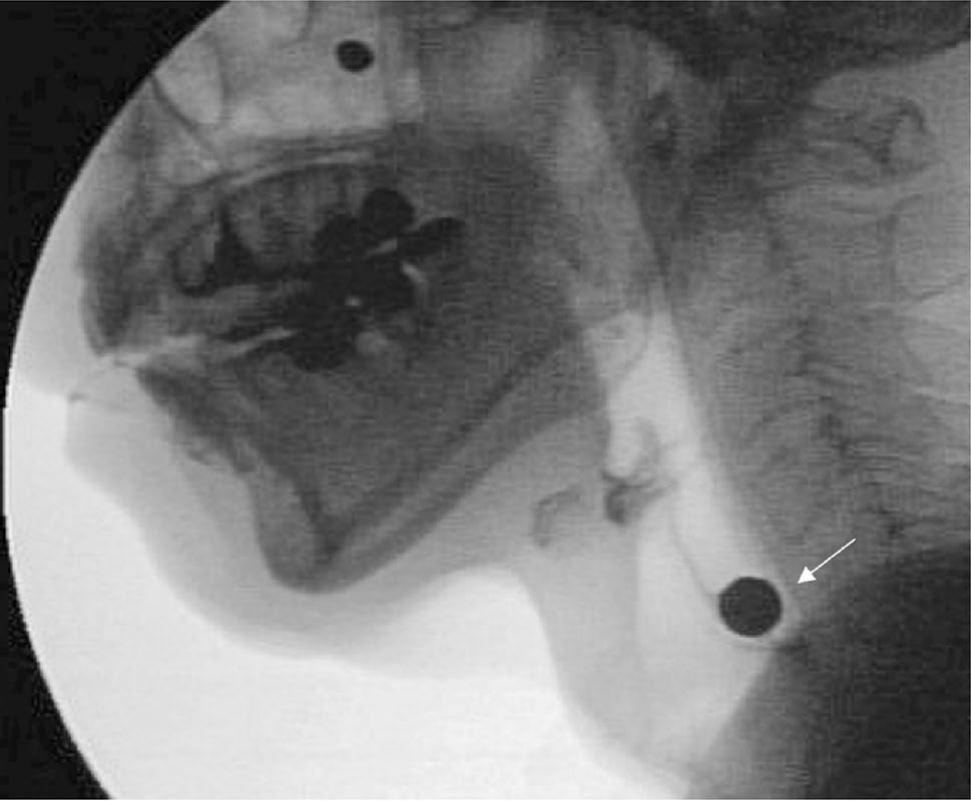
Radiograph, lateral view, showing pill residue in the piriform sinus. The arrow indicates the pill residue.
